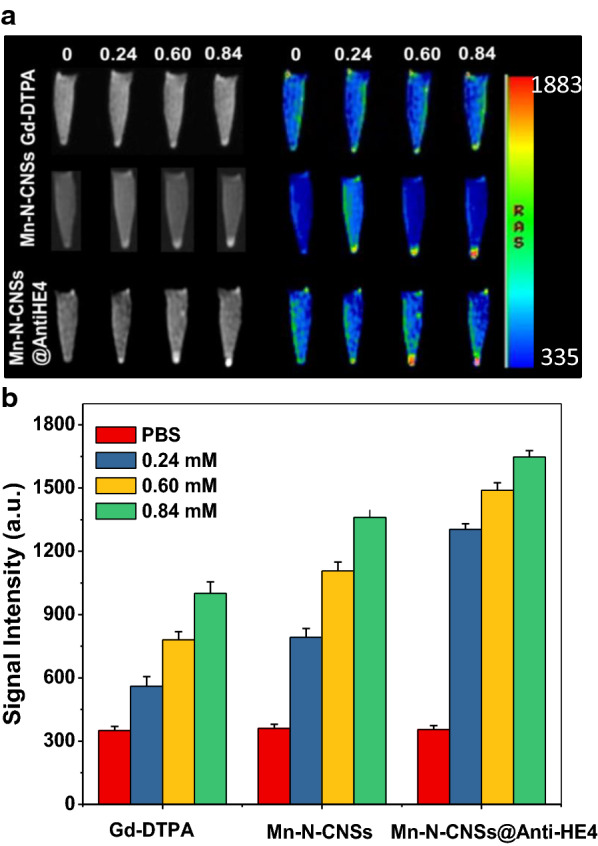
In vitro MR imaging of HO-8910 cells after treated with Gd-DTPA, Mn-N-CNSs and Mn-N-CNSss@Anti-HE4 at various concentrations: a T_1_-weighted and T_1_-map images, b MR signal intensities
